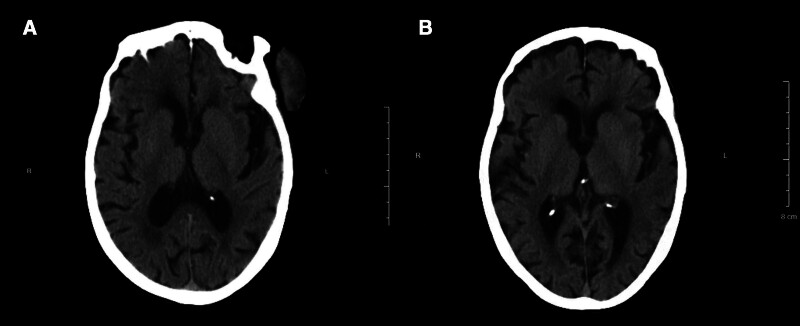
CT scan of the orbit. (A) CT scan after admission; (B) CT scan 6 mo after tumor resection. CT = computed tomography.
